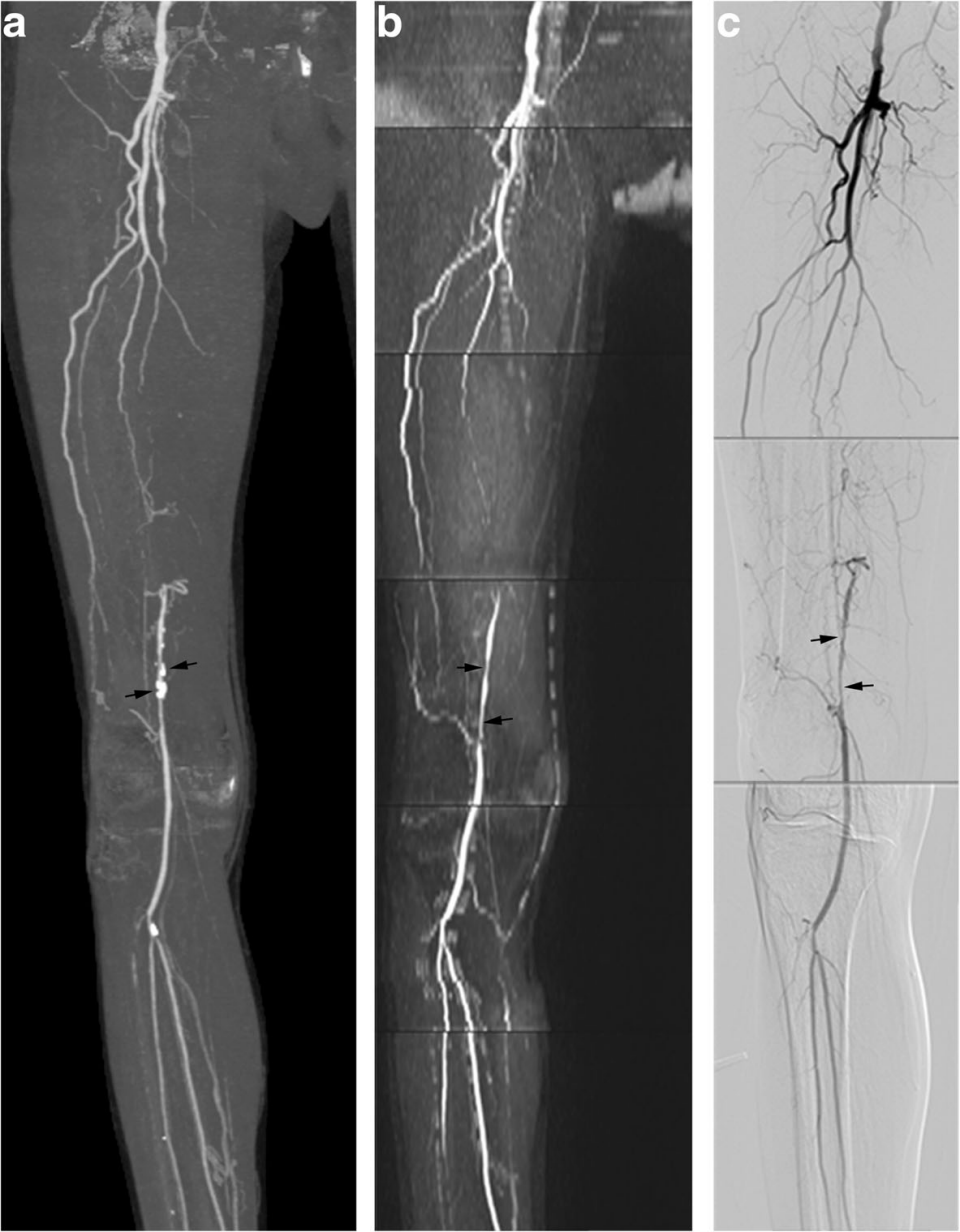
Occlusion of right femoral artery was seen at CTA (a) and QISS MRA (b), and was proved by DSA (c). Stenosis degree is difficult to determine duo to calcified plaques overlapping at CTA MIP image (*arrows*). Significant stenoses (*arrows*) were well seen at QISS MIP image (b) and DSA (c)
